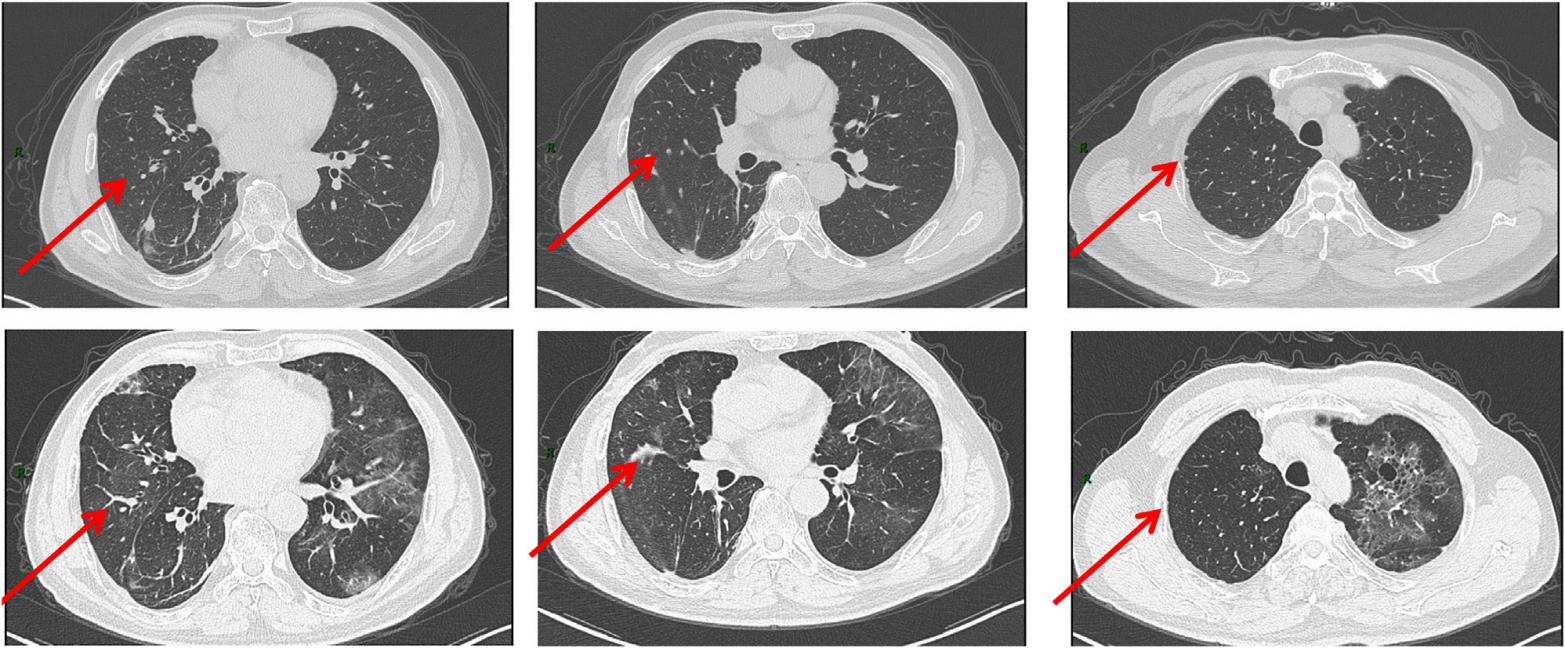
CT images obtained on 2 March 2022(UP) and 5 May 2022 (DOWN). The size of the metastatic lesion was reduced after pralsetinib treatment.
